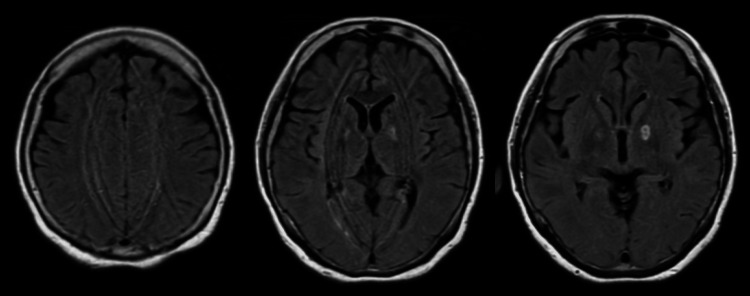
The result of T2-FLAIR brain MRI one month after initial onset. T2-FLAIR brain MRI showing BPL with hyperintensities on T2-FLAIR weighted imaging and no white matter lesion. FLAIR, fluid-attenuated inversion recovery; MRI, magnetic resonance imaging; BPL, bilateral globus pallidus lesions
